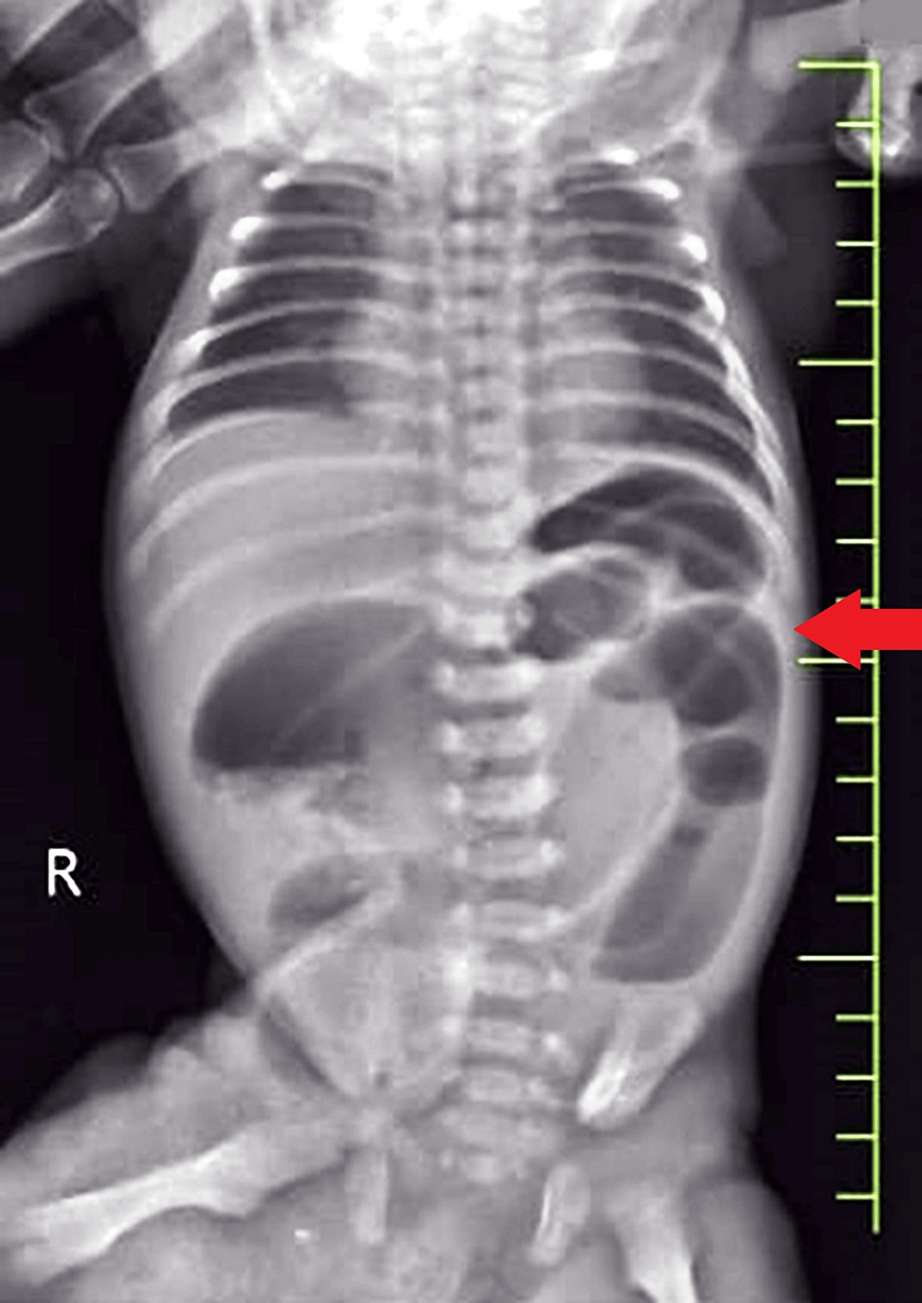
X-ray revealed signs suggestive of intestinal obstruction (red arrow)
